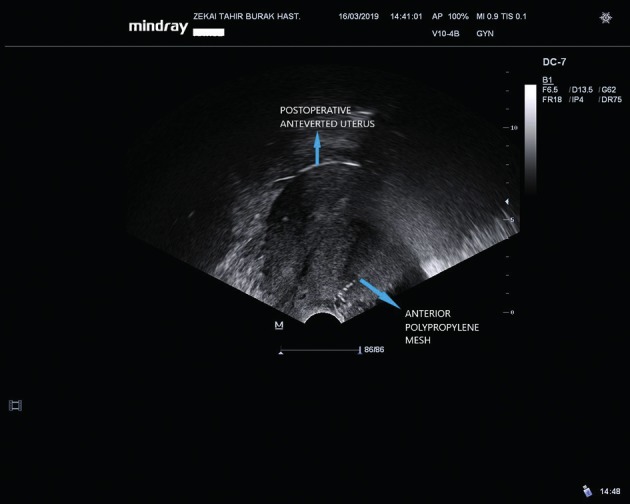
Postoperative transvaginal ultrasonographic view of anteverted uterus and anteriorly anchored polypropylene mesh echogenicity.
